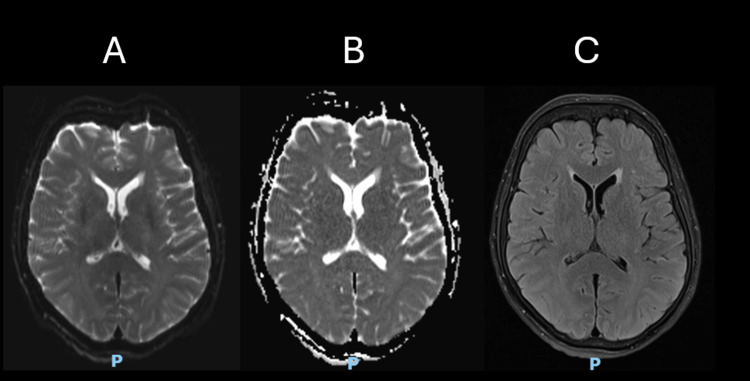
Represents MRI brain post-endovascular embolization axial plane view A) diffusion-weighted imaging, B) apparent diffusion coefficient, C) flair sequences
